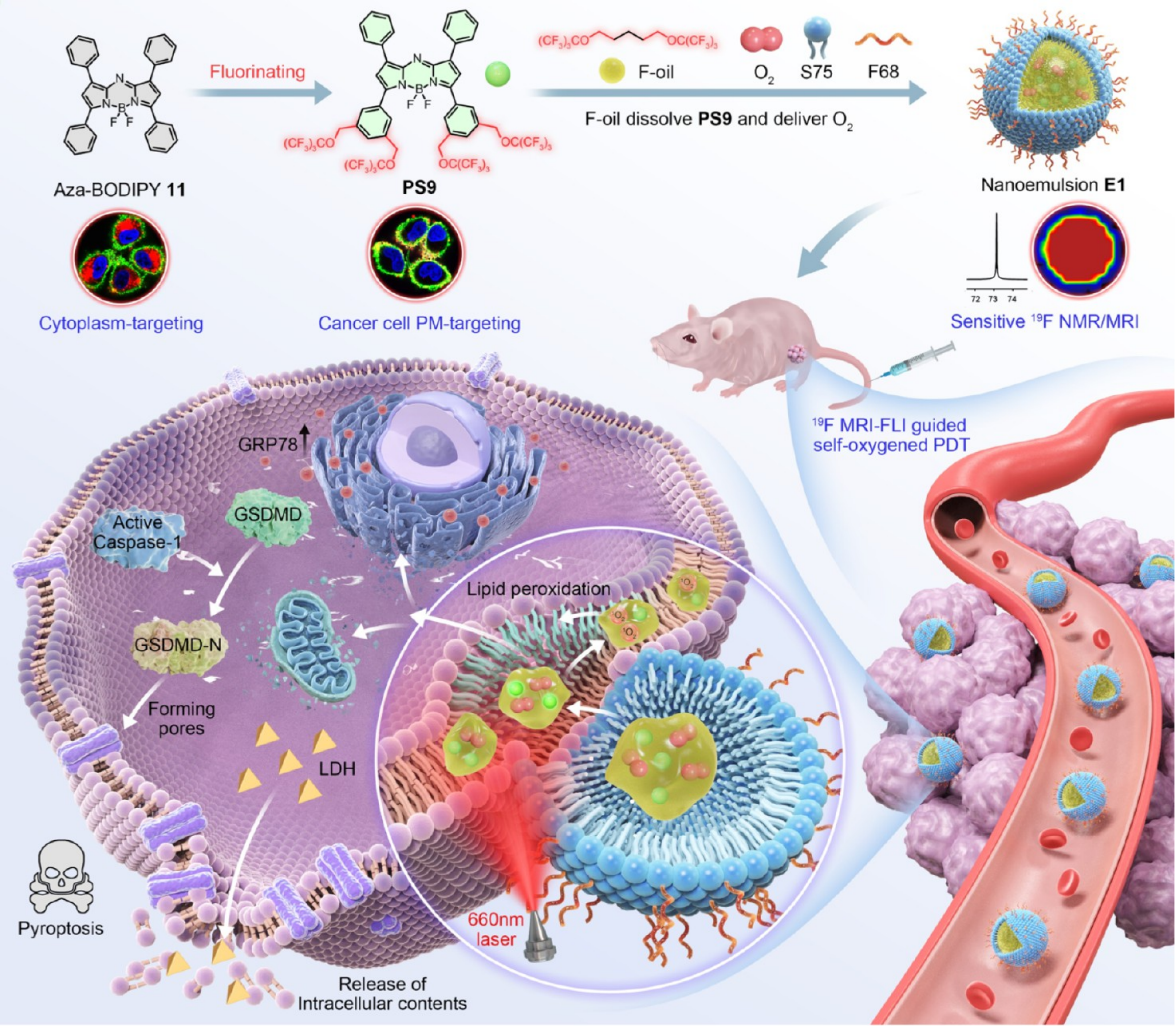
Fluorinating Aza-BODIPY for Cancer Cell PM-Targeted, ^19^F MRI-FLI-Guided, and Self-Oxygenated PDT of Lung Cancer.
